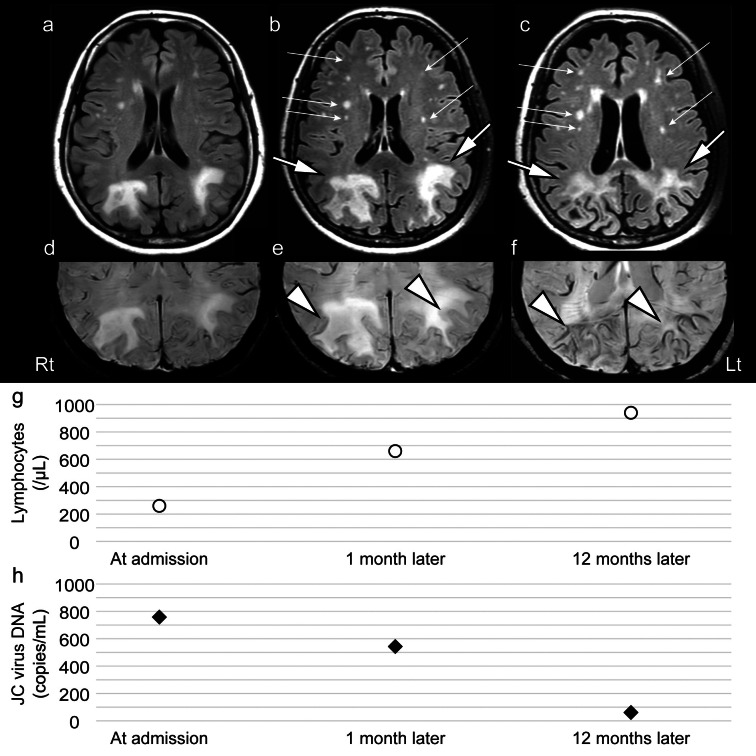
MRI and JC virus DNA copy numbers in the CSF at each time point are shown. Axial FLAIR images a at admission, b one month later, and c 12 months later are shown. SWI findings d at admission, e one month later, and f 12 months later are shown. Lymphocyte number g and JC virus DNA copy number in the CSF h at each time point are summarized. Both large hyperintense and punctate lesions are enlarged on FLAIR images (one month later). After 12 months, the initially large hyperintense lesions on FLAIR images gradually shrank and became pale (large arrows). The punctate lesions on FLAIR images remained prominent (small arrows). SWI shows hypointense signal rims adjacent to white matter lesions in the bilateral parieto-occipital lobe cortex, becoming more prominent over time (arrowheads). The follow-up lymphocyte counts were 660 and 940/μL at one and 12 months. The JC virus DNA copy number in the CSF significantly decreased from 543 to 61 copies/mL after 12 months. Abbreviations: MRI, magnetic resonance imaging; CSF, cerebrospinal fluid; FLAIR, fluid-attenuated inversion recovery; SWI, susceptibility-weighted imaging
